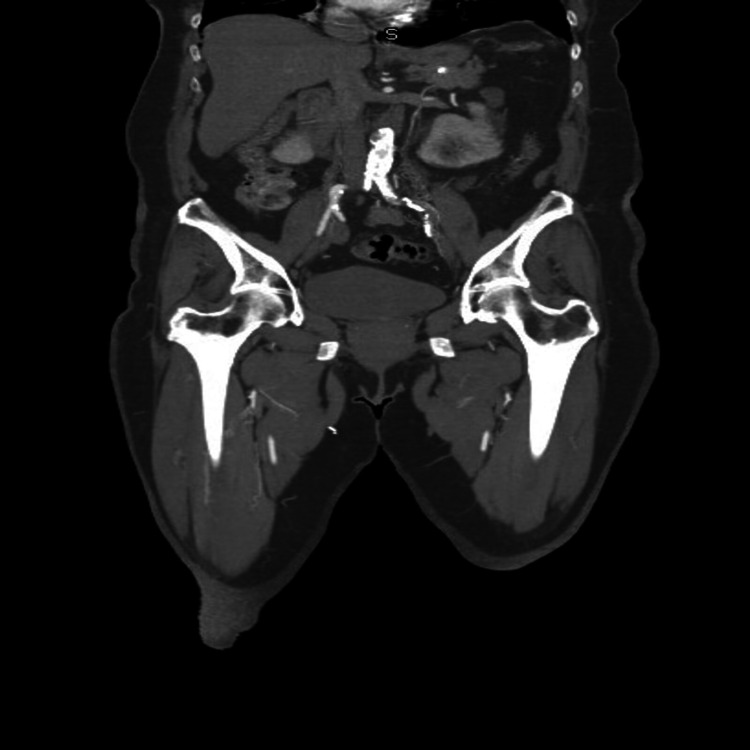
CT of abdomen and pelvis showing right and left iliac artery thrombosis
